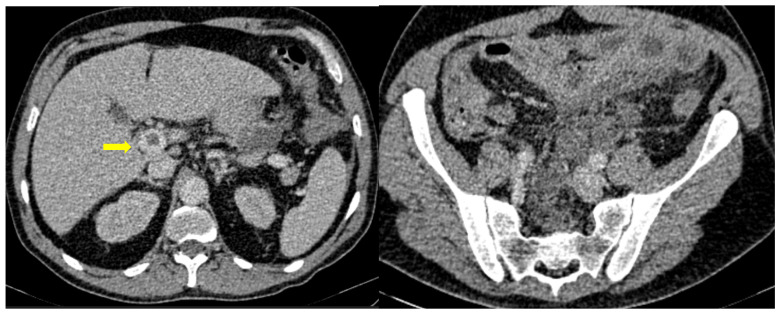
56-year-old hospitalized male patient with COVID-19 pneumonia with raised level of d-dimers. CT depicting the thrombosed portal vein (yellow arrow). Note that in the same scan, there is extended small bowel thickening with mesenteric free fluid.
